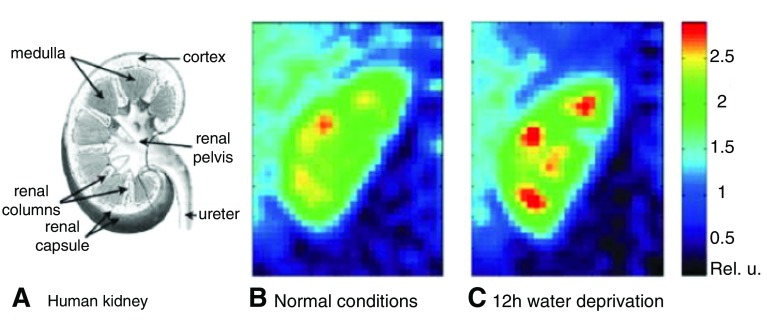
Sodium magnetic resonance imaging (MRI) of the kidney. A) A scheme of the human kidney.
